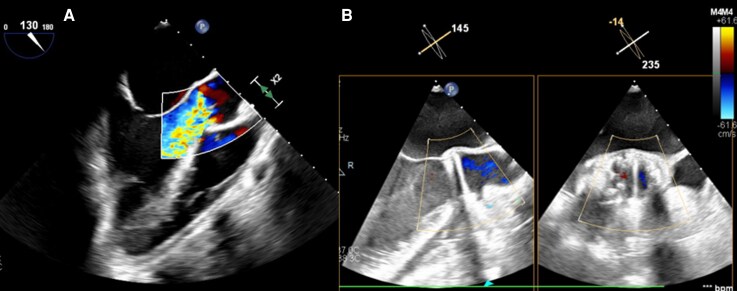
Preoperative severe aortic regurgitation and postoperative mechanical aortic valve replacement. (*A*) Preoperative transoesophageal echocardiography demonstrating severe aortic regurgitation on colour Doppler, consistent with progression of valvular dysfunction. (*B*) Postoperative transthoracic echocardiography demonstrating a mechanical aortic valve prosthesis following surgical aortic valve replacement.
